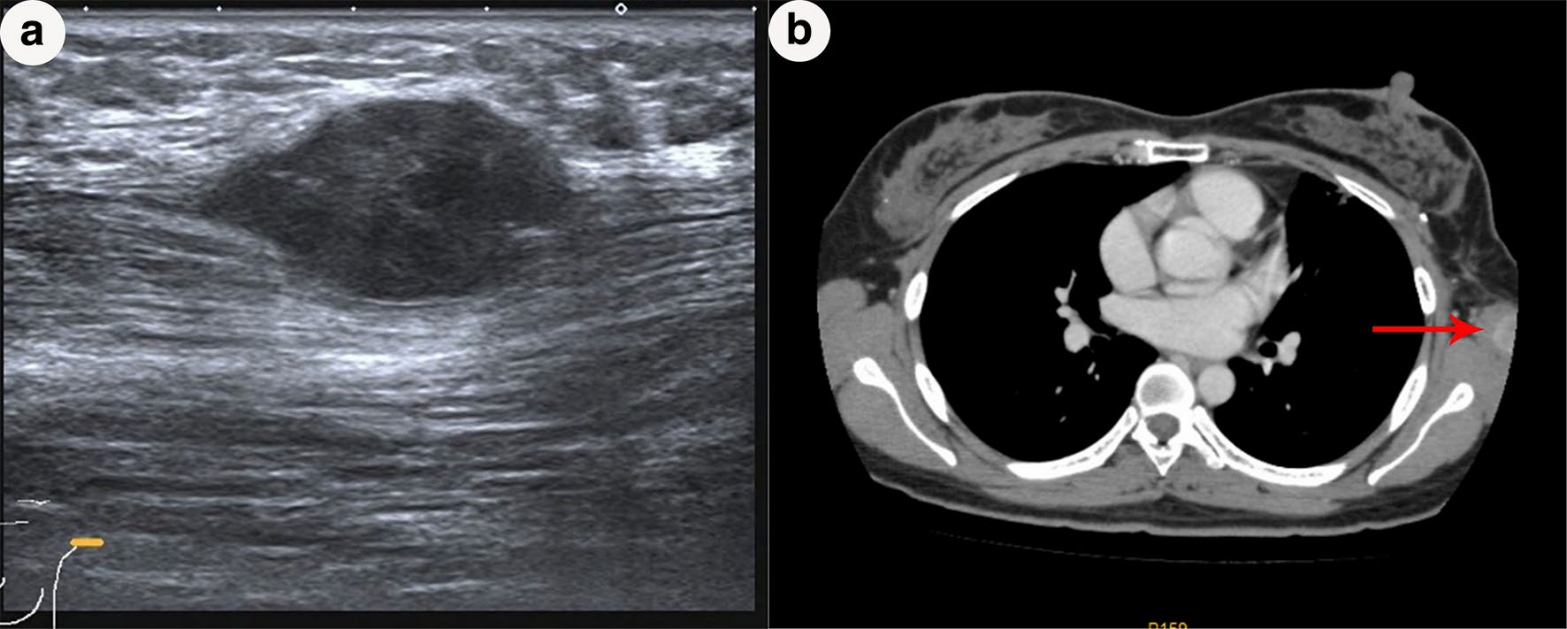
The mammary gland ultrasound showed a solid mass of the chest wall below the left axilla (a); chest CT showed a low-density shadow in the anterior part of the left latissimus dorsi, and the enhanced scan was significantly enhanced (arrow, b)
